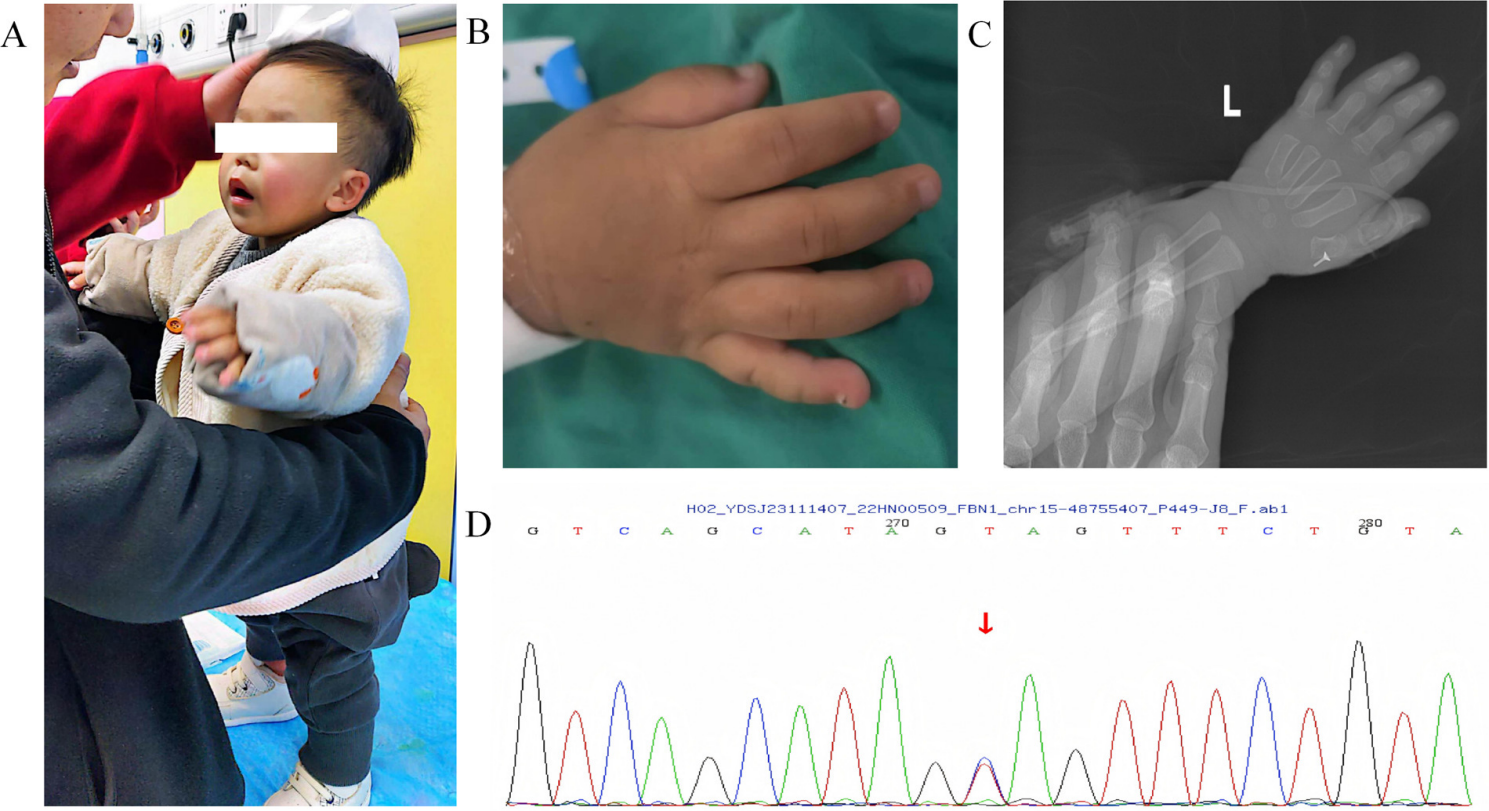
The patient had GD-related symptoms and genetic mutations. (A-B) the patient present with disproportionate dwarfism, short limbs, short arms and legs, (C) Skeletal x-ray showed delayed bone age and epiphyseal dysplasia, (D) whole-exome sequencing revealed *de novo* heterozygous mutations in the patient's FBN1 gene.
